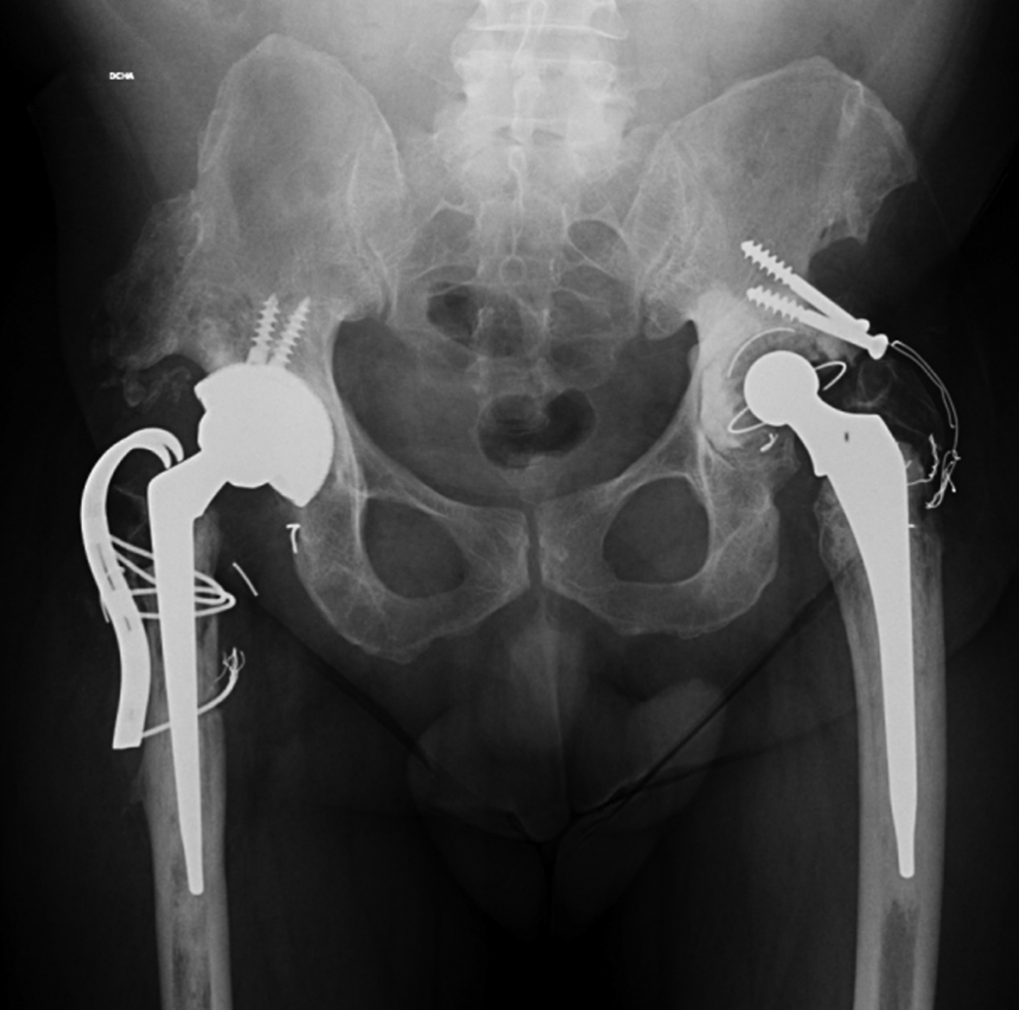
Anteroposterior radiograph of the pelvis where eccentric placement of the metal head can be seen. Signs of failure of the greater trochanter synthesis were also present.
